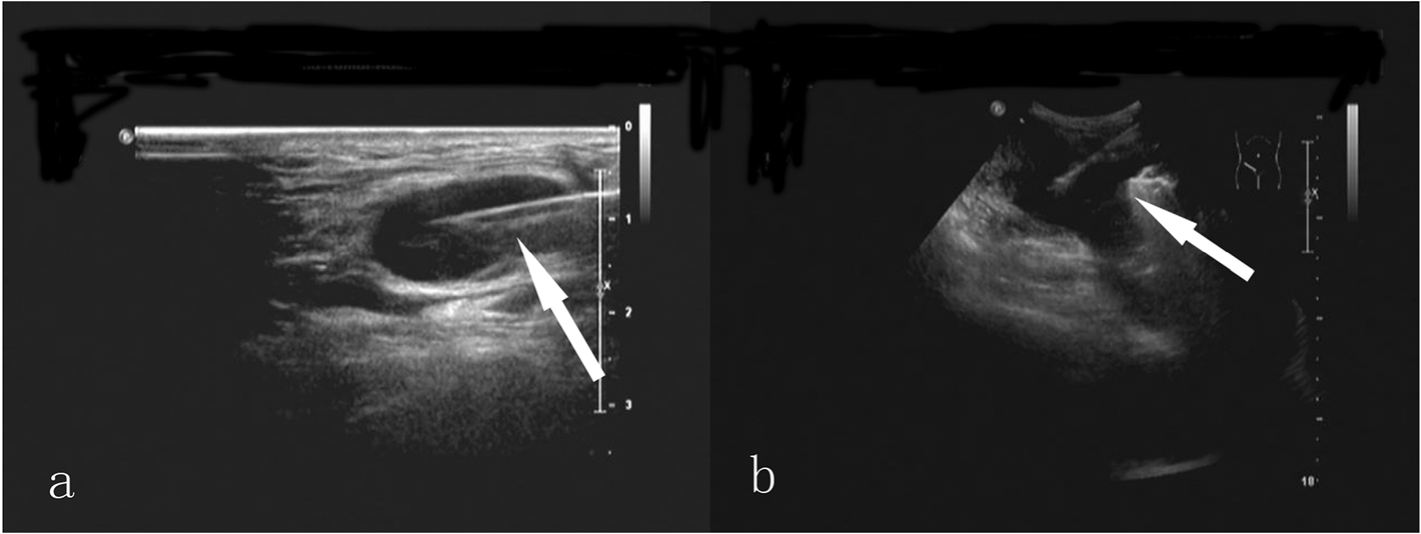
Ultrasonograms of the needle tracts. (a) The ultrasonogram of the needle tract in the superficial masses after ejection of biopsy gun (arrow); (b) the ultrasonogram of the needle tract in the deep masses after ejection of biopsy gun (arrow).
